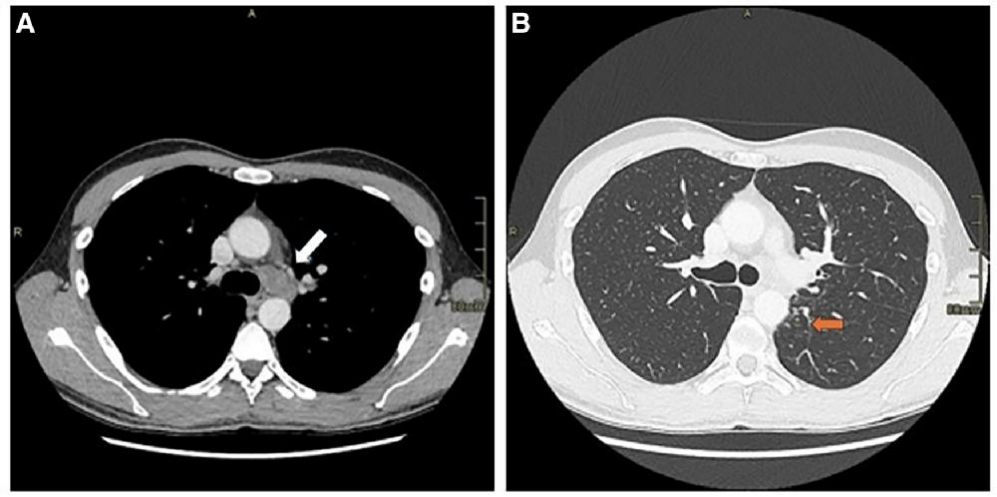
CT scan after 1 year showing the reduction of the mass's dimensions (*A*, indicated by the arrow) and the disappearance of the thickening area in the left pulmonary upper lobe (*B*, indicated by the lower right arrow).
